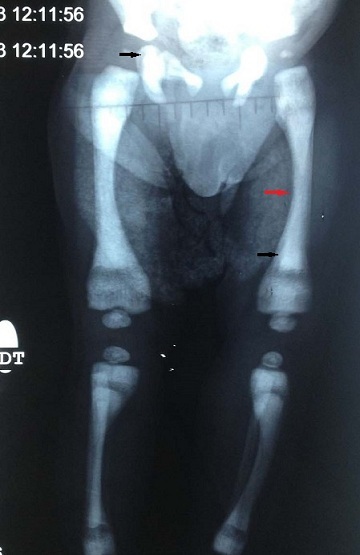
Pelvis and lower limbs radiograph. Note generalized bone density with bone in bone appearance (black arrows) and bowlegs (red arrow)
